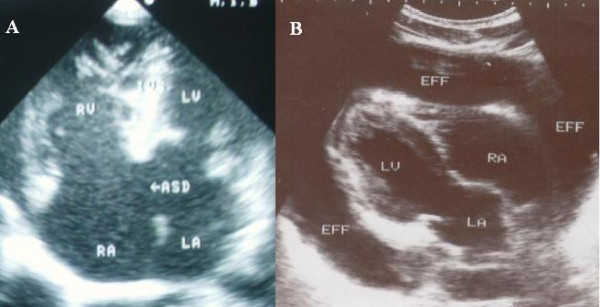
A. echocardiogram of a subject with a large atrial septal defect; B. 2D echocardiogram of a subject with effusive pericarditis.
